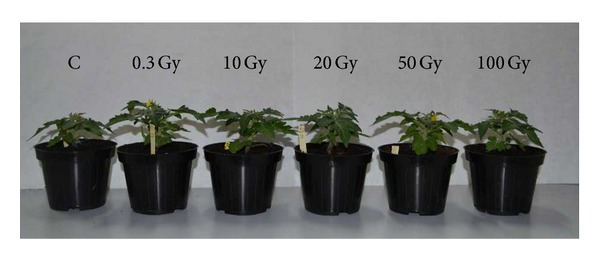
Plants of* S. lycopersicum* L. “Microtom” developed from control and irradiated seeds at 40 DAS (Days After Sowing). Plants from seeds exposed to increasing doses of X-rays (from 0 to 100 Gy) are shown from left to right.
